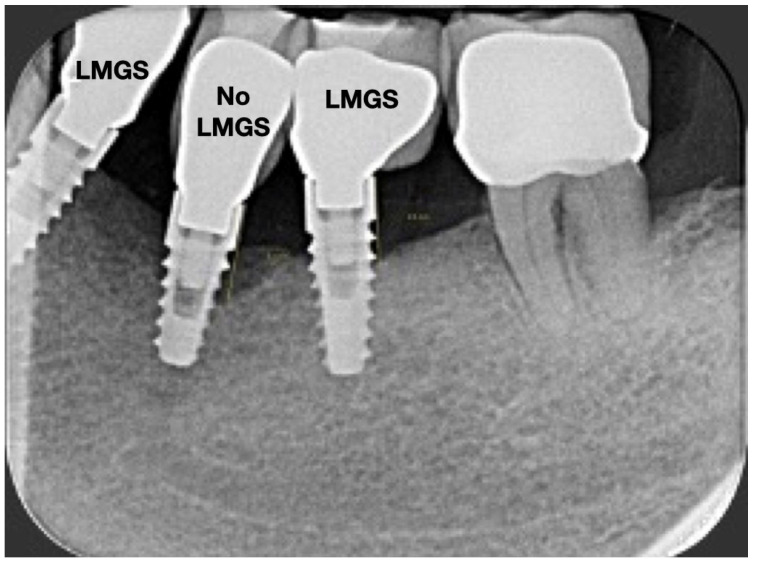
Radiograph of the same three (3) implants at the 15-year follow-up. Signs of P can be noted around the No-LMCS implant. (LMGS = with a laser-microgrooved collar surface; No-LMGS = without a laser-microgrooved collar surface).
